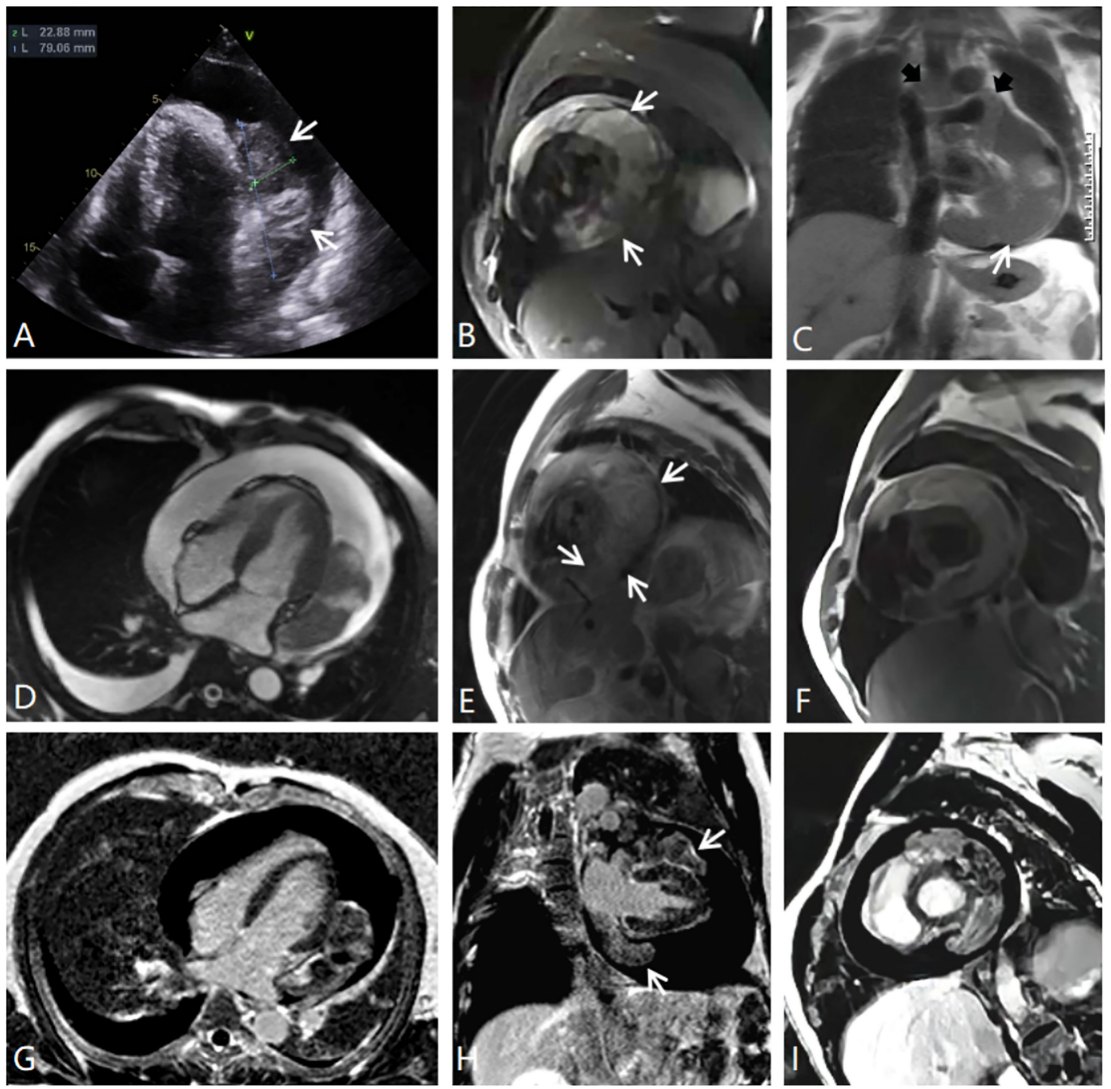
(A) Echocardiography. (B) MRI fat suppression image, short axial position of the left ventricle. (C) Half-Fourier single-shot turbo spin-echo MRI coronal image showing the tumor-encased superior vena cava, ascending aorta, and pulmonary trunk *(black arrows)*. (D) MRI cine sequence, four-chamber position. (E) MRI T2-weighted image, short axial position. (F) MRI T1-weighted image, short axial position. (G–I) MRI delayed enhancement, four-chamber position, two-chamber position, and short axial position. There is an inhomogeneous, enhancing intrapericardial mass surrounded by fluid, with no intracavitary or endoluminal invasion.
